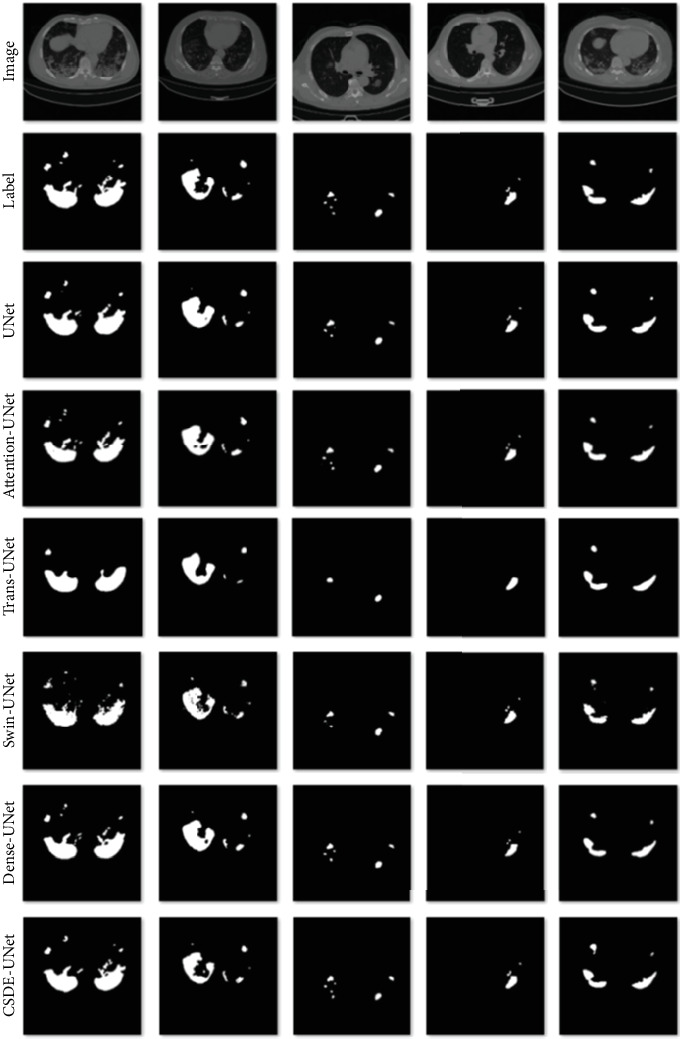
The segmentation comparison of CDSE-UNet with other counterpart models on five CT images.
